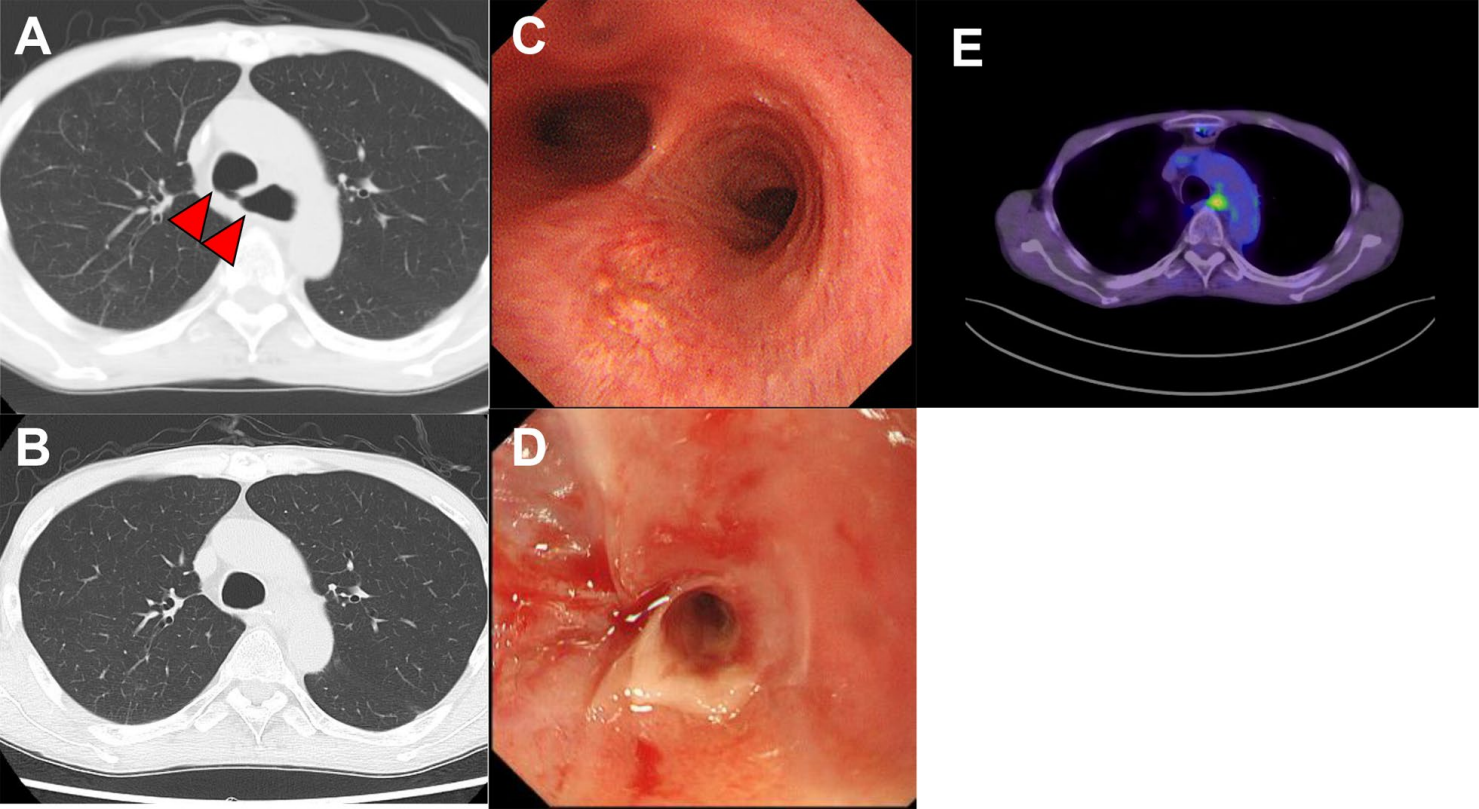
Examinations after definitive CRT. A, B CT images. Continuity between the trachea and esophageal lumen (arrowheads) was observed, suggesting esophagotracheal fistula. B CT after treatment for esophagotracheal fistula. The continuity disappeared. C Bronchoscopy. No fistula or tumor exposure were detected. D EGD image. Severe stenosis was developed, which prevented passage through the scope. E FDG-PET-CT after CRT. There was decreased accumulation of FDG in the thoracic esophagus, with no obvious distant metastases
